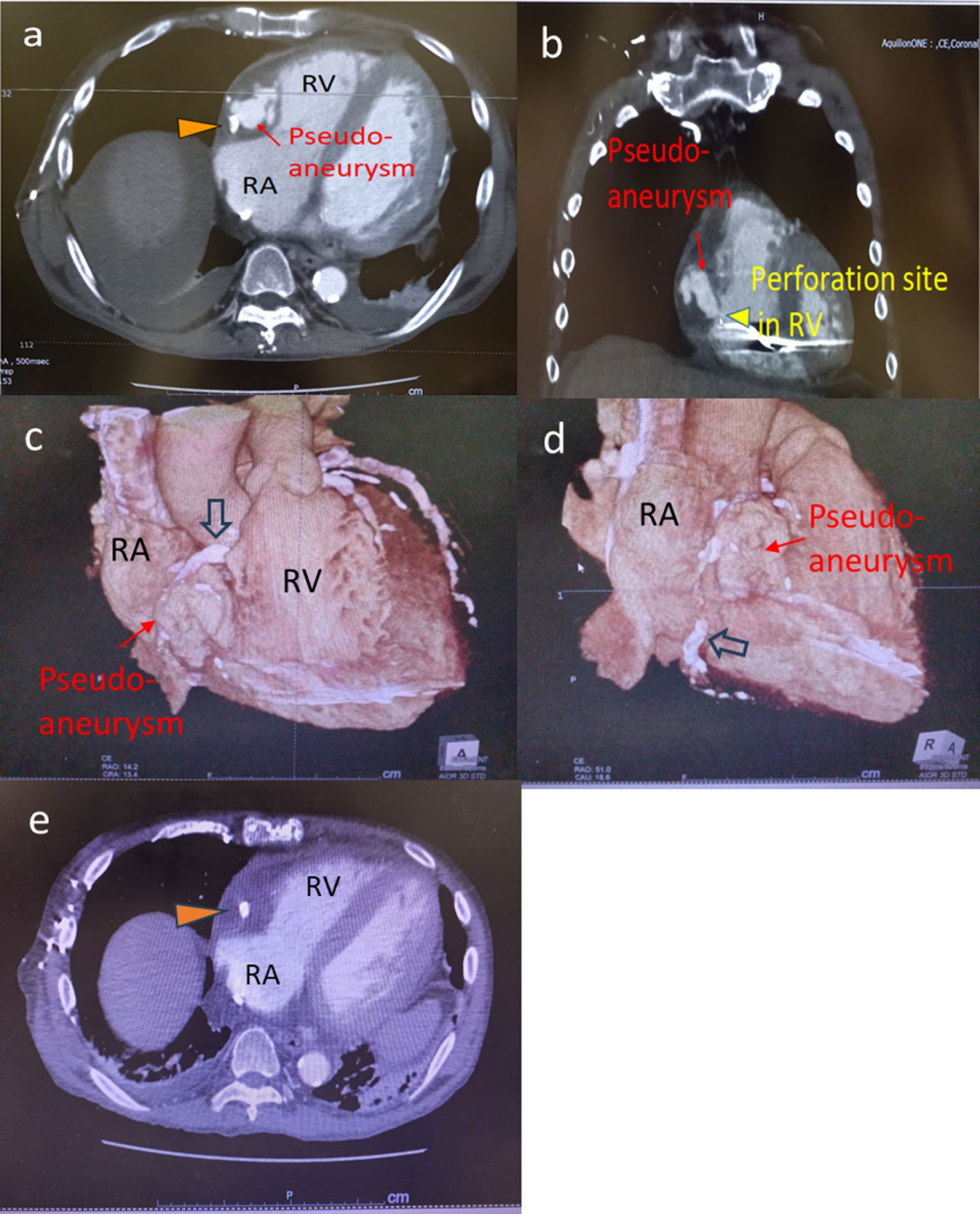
Preoperative enhanced computed tomography. a Axial view showing the right coronary artery (arrowhead) with severe calcification around the pseudoaneurysm (arrow). b Coronal view showing shunt flow to the RV through the pseudoaneurysm (arrow) and the perforated RV (arrowhead). c and d 3-dimension CT showing pseudoaneurysm measuring 5.5 × 4.0 cm around the RCA with severe calcification (open arrow). e Enhanced CT with an axial level equivalent to a on admission. No pseudoaneurysm was seen around the calcified RCA (arrowhead). CT; computed tomography; RA, right atrium; RCA, right coronary artery; RV, right ventricle
